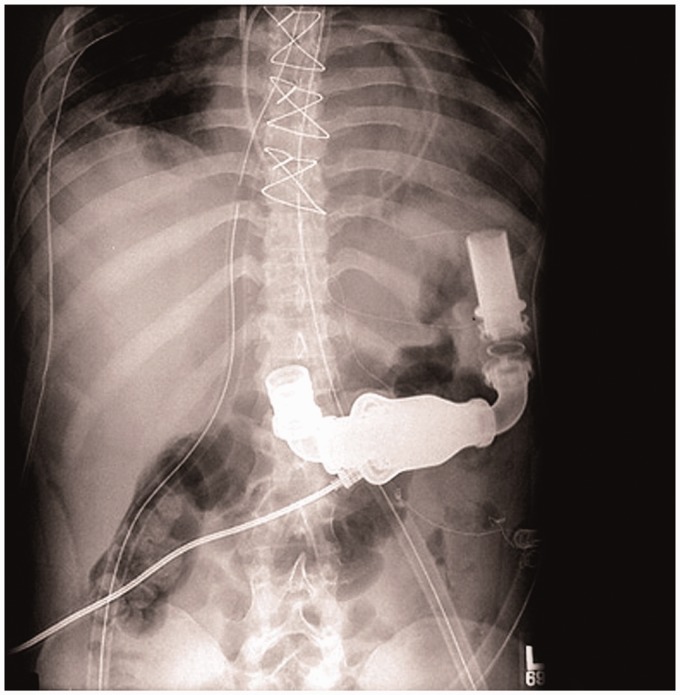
X-Ray of a patient with HeartMate II LVAS (Courtesy of Thoratec Corp.).
